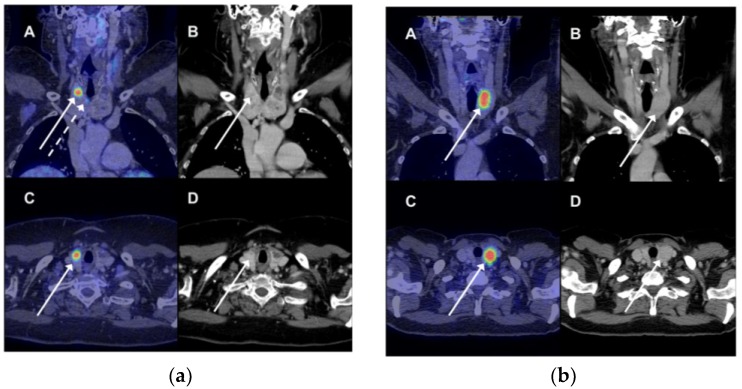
(a) A 69-year woman had a 18F-fluorodeoxyglucose positron emission tomography/computer tomography (FDG PET/CT) scan because of suspected pancreatic cancer (not verified). There was incidental FDG uptake in three lesions in the right thyroid. The patient underwent total thyroidectomy; pathology showed papillary adenocarcinoma in all three PET-avid lesions. (A) Fused coronal PET/CT images showed intense FDG uptake (standardized uptake value, SUV 9.8) in the upper right part of the right thyroid (full white arrow). Another site of pathological FDG uptake was seen on this coronal slice as well (dotted white arrow). (B) The CT image showing a bilateral nodular goiter with an arrow indicating the site of most intense FDG uptake on the fused images. (C + D) Corresponding transverse images of the fused PET/CT images and CT scan with the most intense FDG-avid lesion; (b) An FDG PET/CT was performed in a 69-year woman due to suspicion of ovarian cancer (confirmed). Incidental FDG uptake was seen in the left lobe. A fine needle aspirate showed benign findings (colloid nodule). Any false-negative findings were ruled out during clinical and imaging follow. (A) Fused coronal PET/CT images showed intense FDG uptake (SUV 11.0) in the upper right part of the right thyroid (full white arrow). (B) The CT image showing a bilateral nodular goiter with an arrow indicating the most intense site of pathological FDG uptake on the fused images. (C + D). Corresponding transverse images of the fused PET/CT images and CT scan of the most intense FDG-avid lesion.
